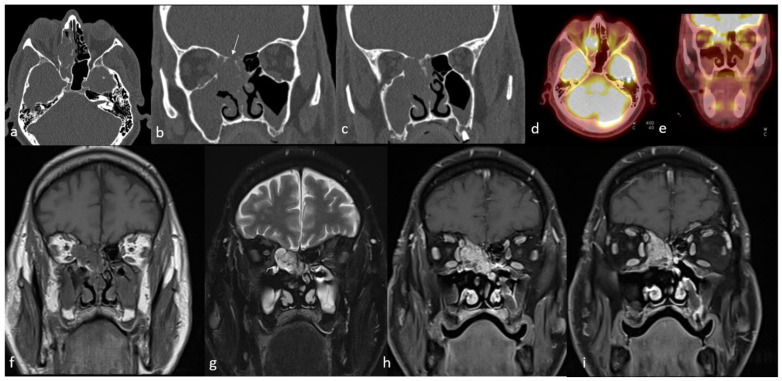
Sinonasal adenocarcinoma. A 62-year-old man with a history of chronic sinus disease presenting with right-sided epistaxis. Initial CT (a–c) shows an expansile right nasal cavity mass centered in the superior/supreme meatus as well as involving the right middle turbinate. Right extraconal extension through lamina papyracea dehiscence is present. There is also dehiscence along the cribriform plate (arrow, (b)). Axial (d) and coronal (e) fused ^18^F-FDG PET/CT images demonstrate a hypermetabolic right ethmoid sinus mass with no evidence of lymphadenopathy or distant metastatic disease. Coronal T1w (f), T2w (g), and post-contrast T1w (h) show a heterogeneously enhanced right ethmoid sinus lesion with extraconal extension. The more anterior post-contrast T1w (i) image does not demonstrate abnormal leptomeningeal enhancement despite the underlying bone dehiscence. Subsequent pathology showed an intestinal type of adenocarcinoma with a mucinous growth pattern.
